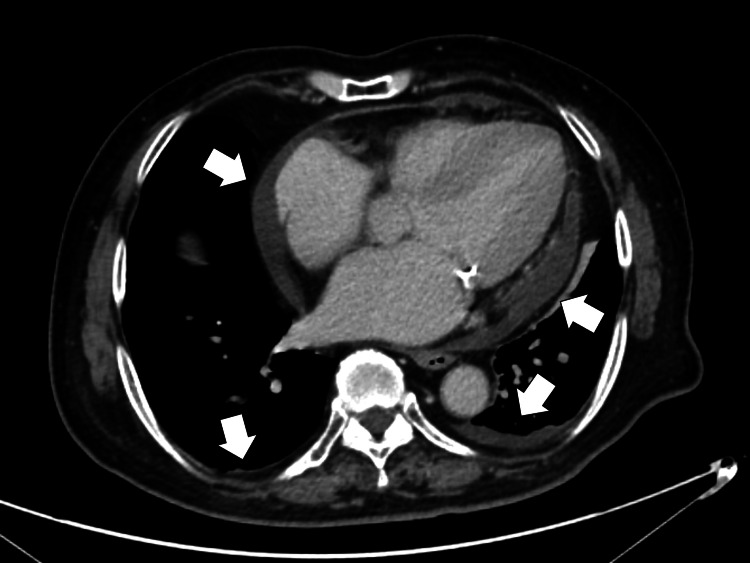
Chest computed tomography with contrast showing bilateral pleural and pericardial effusions (white arrows)
